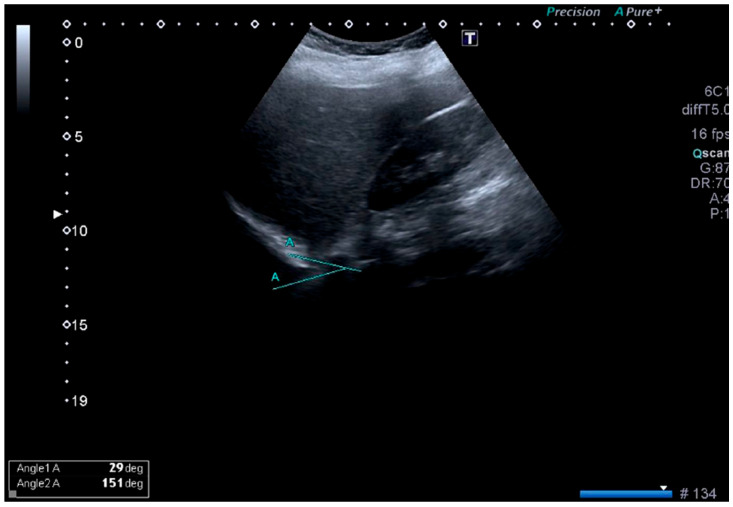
Ultrasonographic measurement of the costophrenic angle (CPA). Representative ultrasound image demonstrating the angle used for CPA assessment.
